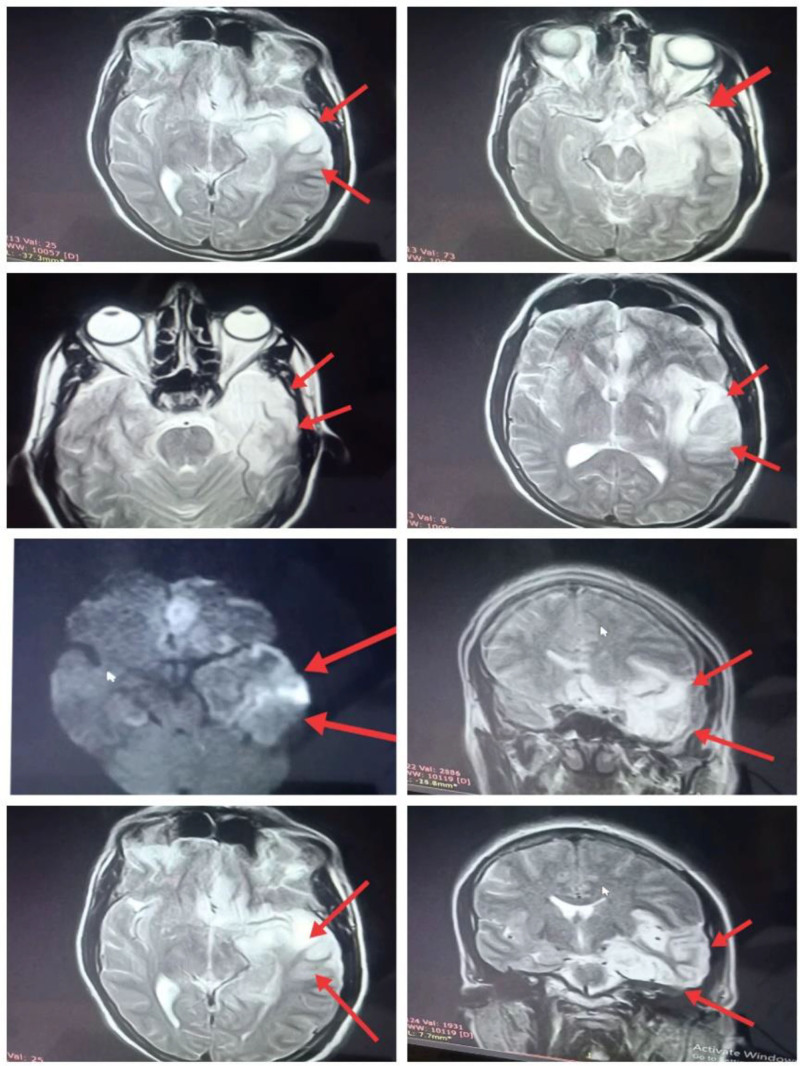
Axial, coronal, and sagittal magnetic resonance brain images on T2-weighted and FLAIR sequences showing hyperintense lesions in the temporal lobe, predominantly involving the medial region.
